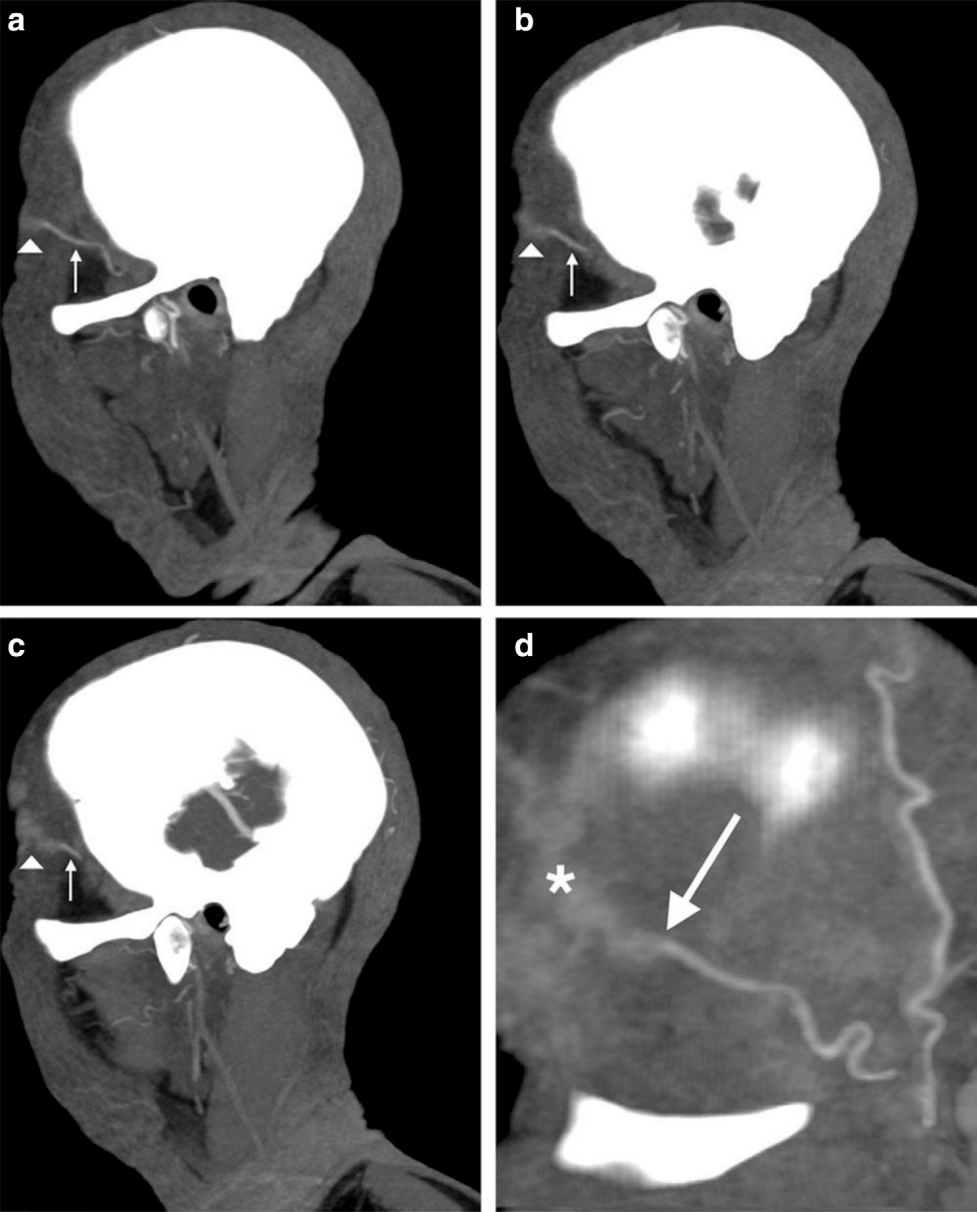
Cigar smoke sign on sagittal maximum intensity projection CTA images from a 71- year-old male with GCA. (a–c) Left superficial temporal artery is depicted with a normal proximal segment, with less well-defined distal segment, represented by the arrow and arrowhead, respectively. (d) Magnified view of blurred soft-tissue enhancement in the distal left superficial temporal artery. Reproduced from Conway, R., Smyth, AE, Kavanagh, RG, et al, Diagnostic Utility of Computed Tomographic Angiography in Giant Cell Arteritis, Stroke, 49, 9, by permission of Wolters Kluwer Health, Inc. CTA, CT angiography; GCA, giant cell arteritis
